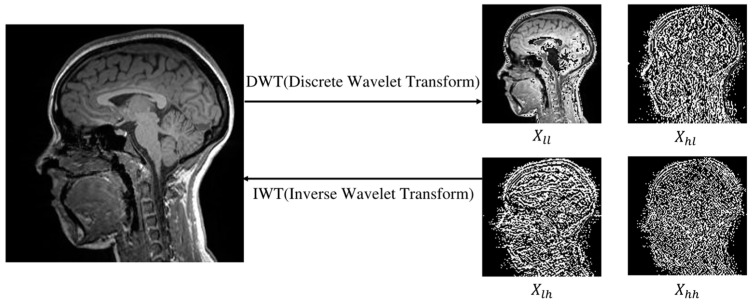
Visualization of the low-frequency component ($X_{ll}$) and high-frequency components ($X_{hl}$, $X_{lh}$, $X_{hh}$) decomposed from MRI images using a wavelet transform. The high-frequency component represents the edge part, and the low-frequency component represents the body part, so that they can be used as the diffusion conditions, capturing the main information of MRI images while saving time.
